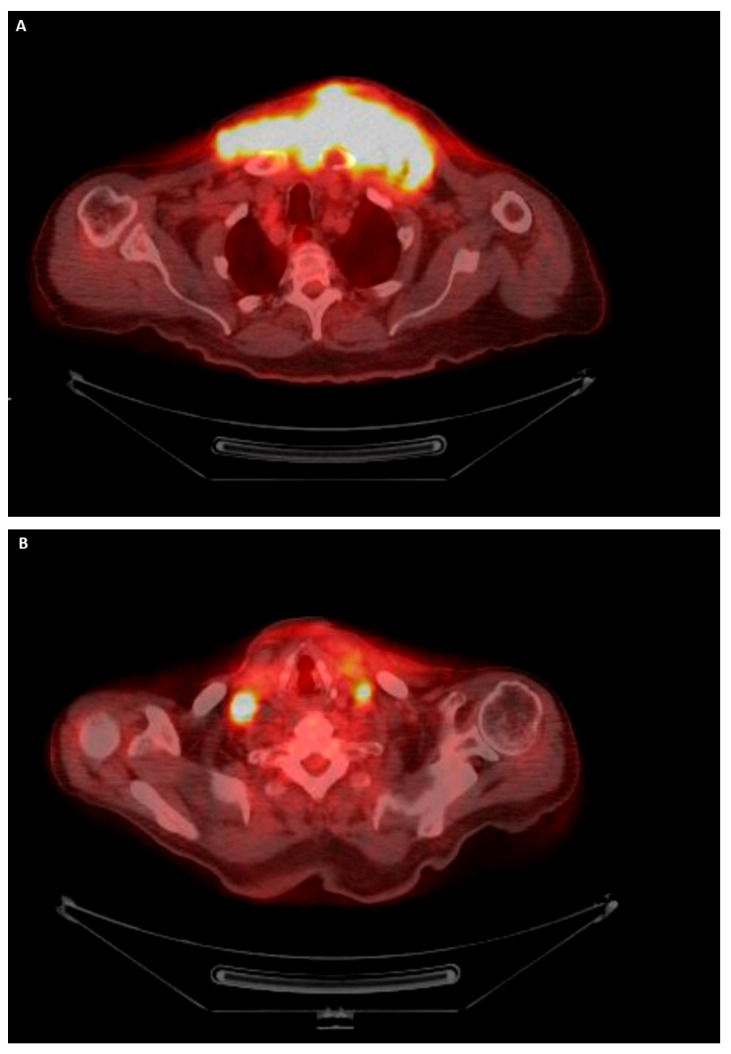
PET/CT imaging of the whole of the body. (A) Mediastinal mass. (B) Lymphadenopathy.
